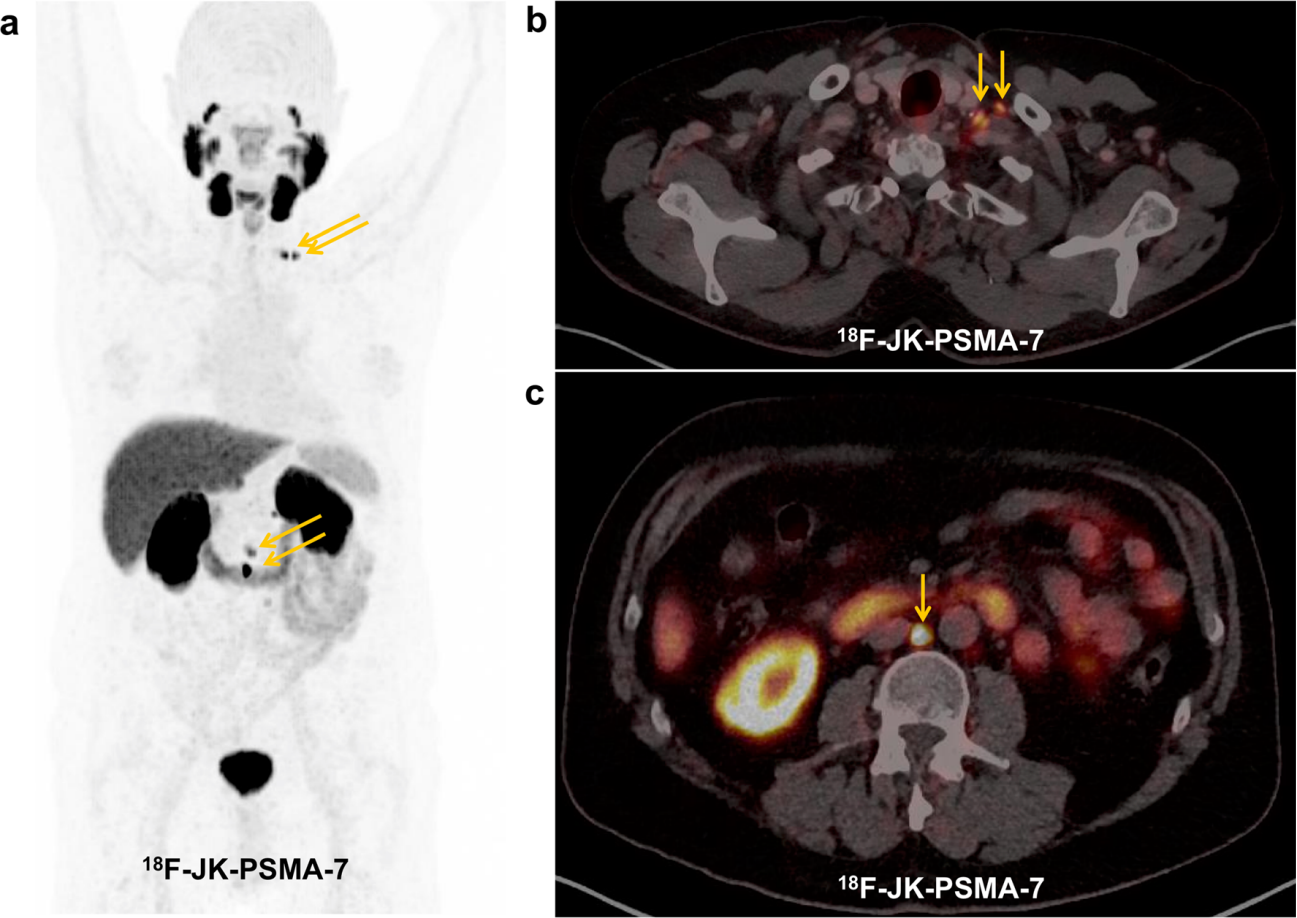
a, b, c The [^18^F]-JK-PSMA-7 PET/CT shows PSMA-positive lymph node metastases left cervical and retroperitoneal despite a low PSA level < 0.1 ng/ml under ADT.
